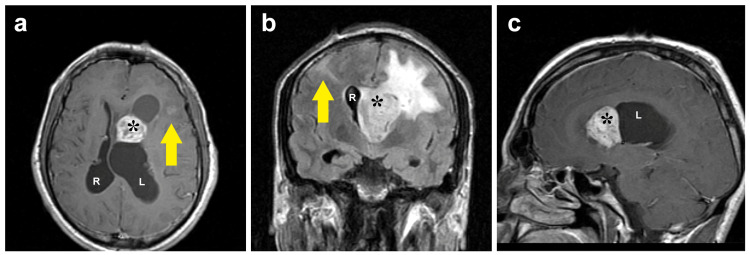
Magnetic resonance imaging of the brain in this case. The images show (a) transverse, (b) coronal, and (c) sagittal sections of the T1-weighted magnetic resonance imaging (MRI) of the brain with gadolinium enhancement showing a 2.5×2.8×3.7 cm lesion on the lateral and frontal wall of the left cerebral ventricle (asterisk). Note also the enlarged left ventricle (L), the midline deviation with the compressed right ventricle (R), and the cortical tubers (arrows).
